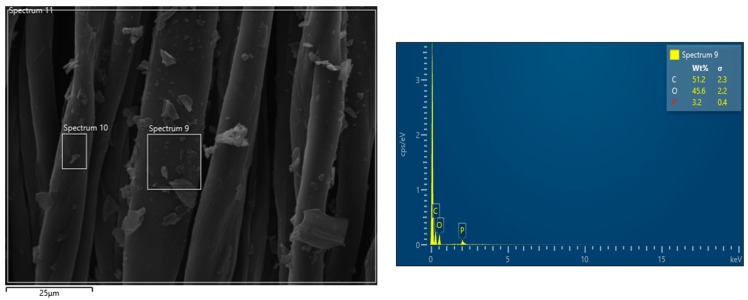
Energy dispersive X-ray (EDX) mapping of COT+PA+BC(8).
